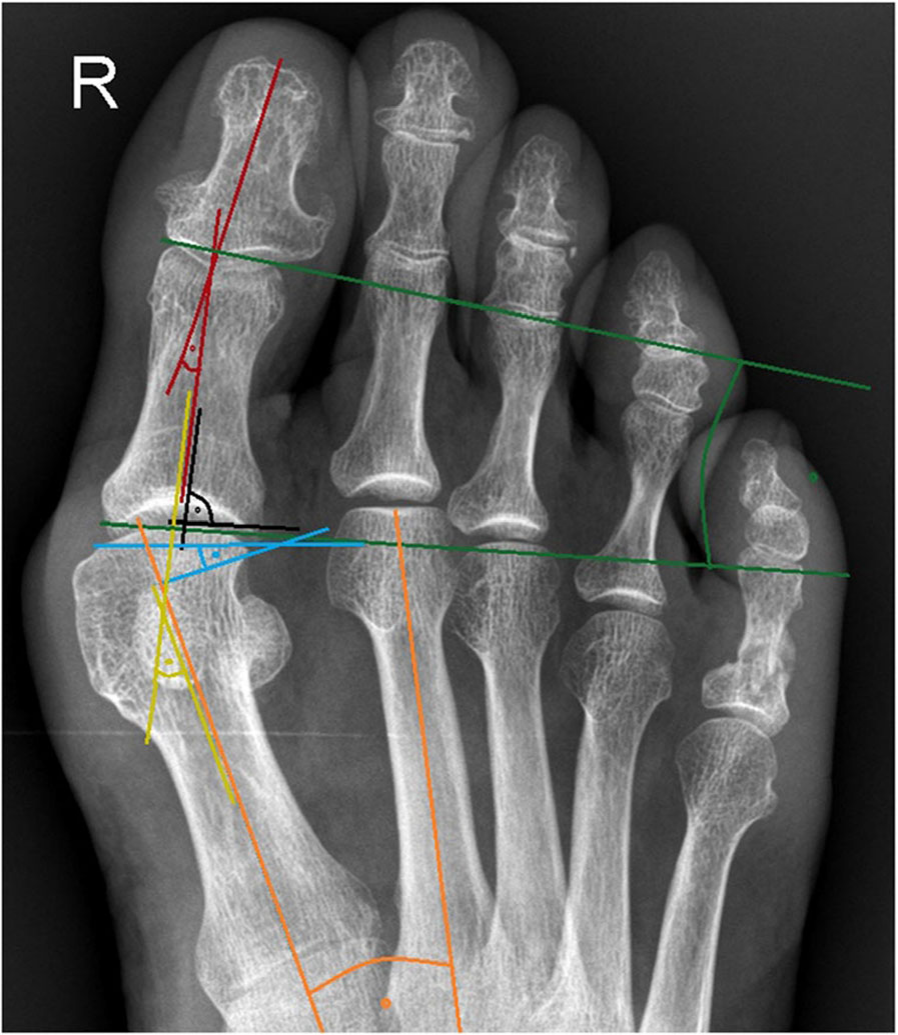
Anteroposterior radiograph showing all relevant angles used to define deformity: HVA (yellow lines), IMA (orange lines), DMAA (blue lines), and PDPAA (green lines)
